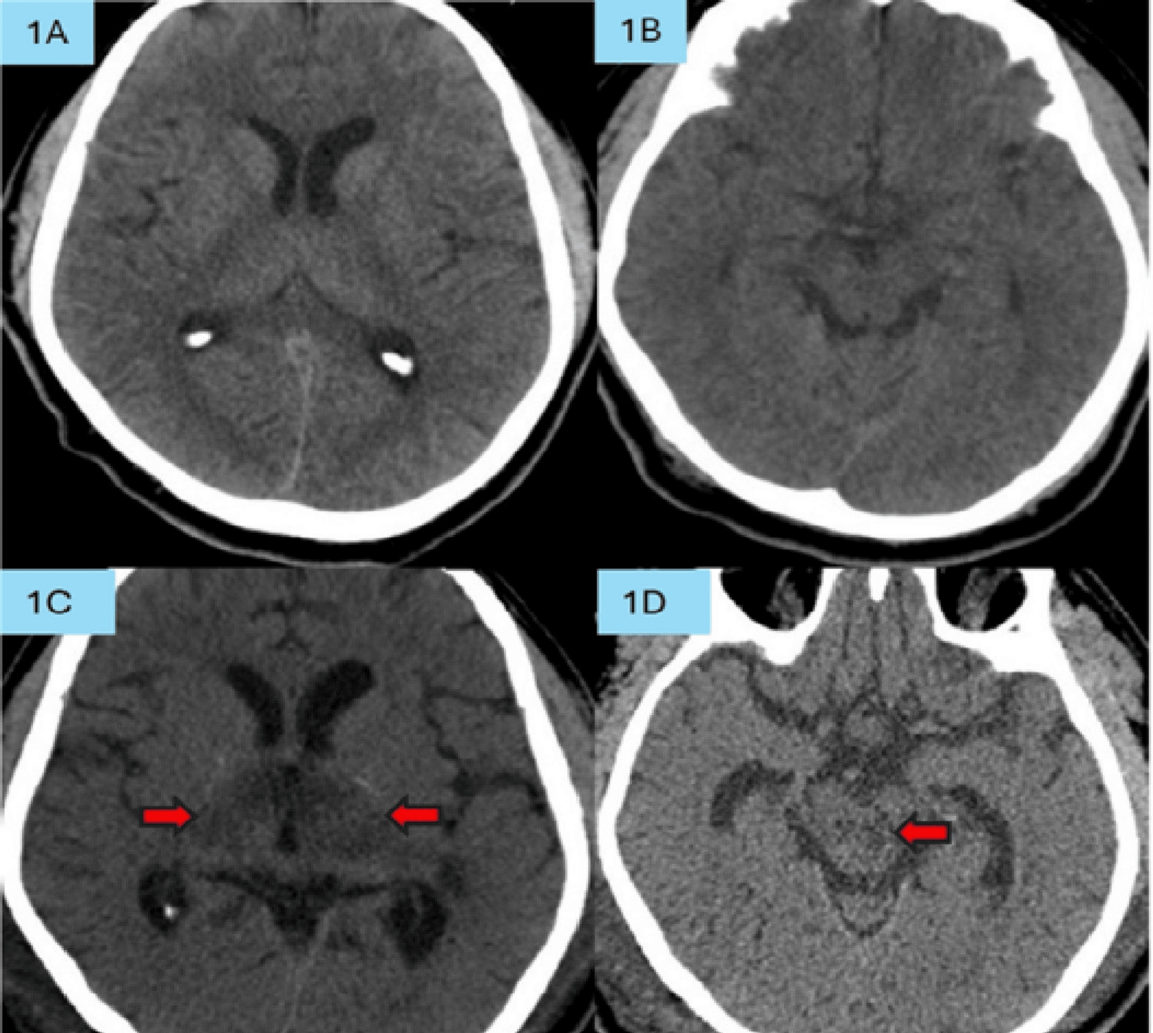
The initial brain CT scans (Figures 1A and 1B) show no evidence of infarction in the thalamus or midbrain. On repeat brain CT imaging (Figures 1C and 1D), new infarctions are noted, indicated by red arrows, involving the thalamus (Figure 1C) and the midbrain (Figure 1D).
